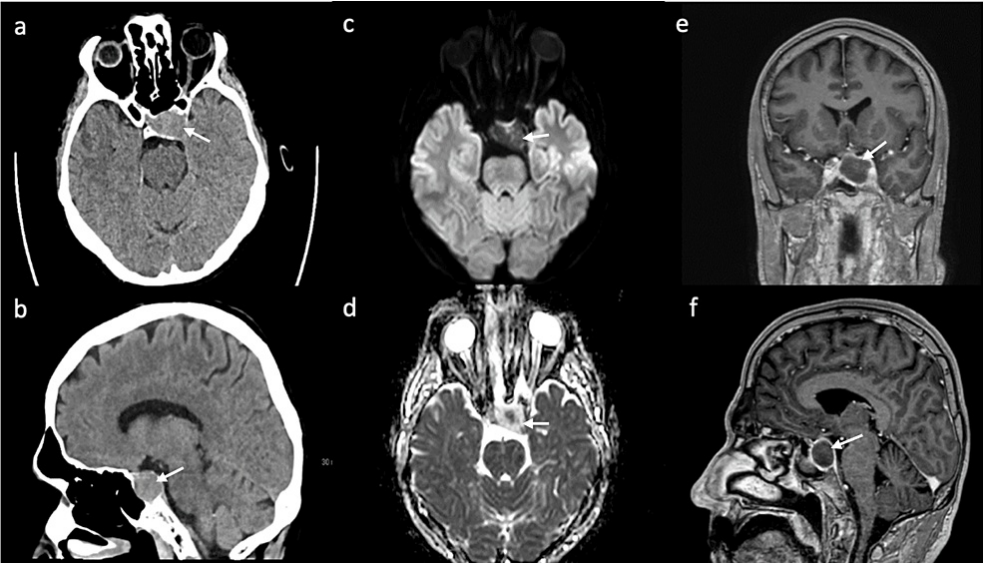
Initial unenhanced CT showing a sellar mass (arrow) with cavernous sinus infiltration of intermediate density in axial (a) and sagittal (b) images. MRI was performed two days later. Diffusion-weighted axial images show a central area of low ADC (d) but otherwise no diffusion restriction in the mass (c). Contrast enhanced T1-weighted images show marginal enhancement of the mass in the coronal (e) and sagittal (f) images. CT: computed tomography, MRI: magnetic resonance imaging, ADC: apparent diffusion coefficient.
